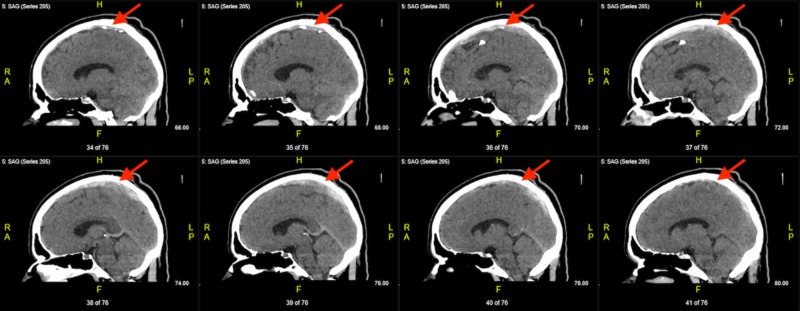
Serial sagittal non-contrast computed tomography (CT) demonstrating a left-to-right progression of sinuses with thrombus
